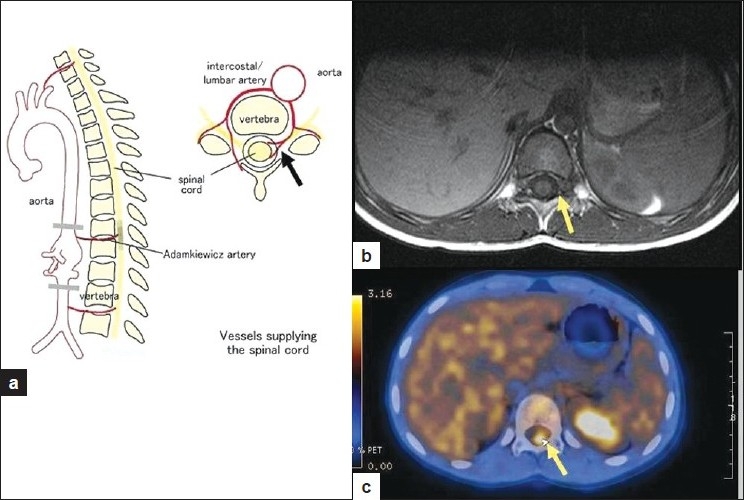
a) Showing pictorial representation of vessels supplying Spinal cord in sagittal and transaxial views. The Adamkiewicz artery is the largest segmental medullary artery typically arises from a left posterior intercostal artery, which branches from aorta and supplies two thirds of the spinal cord via anterior spinal artery. In 75% of people, the artery of Adamkiewicz originates on the left side of the aorta between the D9 and D11 vertebral segments. In approximately 30% of people it arises from the right side. 25% of population can have two large anterior segmental medullary arteries; b) MR Transaxial view showing no altered signals in D11/D12 levels; c) Transaxial view of FDG PET CT depicting abnormal FDG uptake intraspinally at D11/12 vertebral level (SUV Max is 3.95 g/ml). The branching of vessels supplying spinal cord have been highlighted with arrow in all three images
